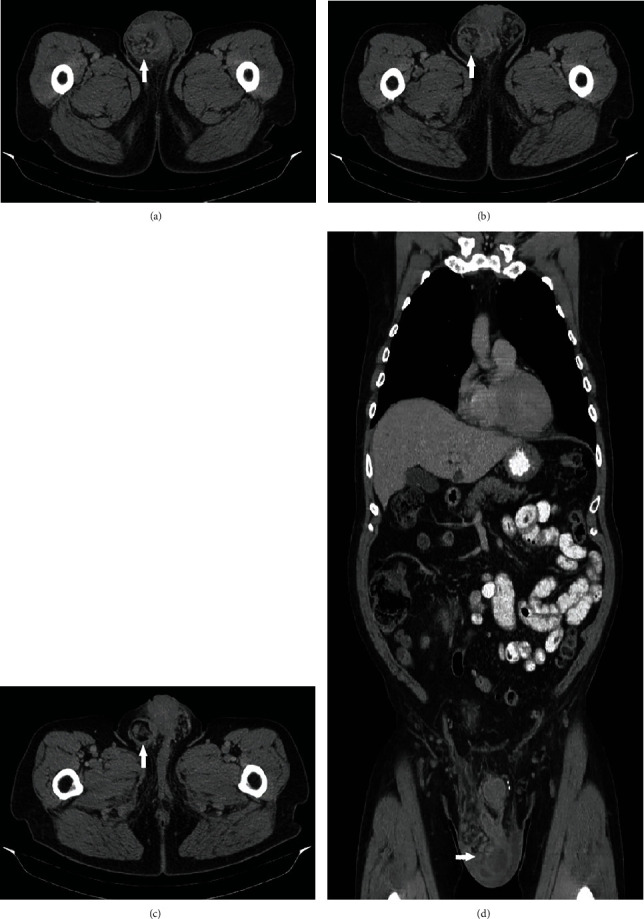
Computed tomography (CT) scan of the chest, abdomen, and pelvis noting a right-sided scrotal mass (white arrows) without signs of a primary tumor site or metastasis. Axial view (a-c) shows the scrotal mass (white arrow), and coronal view (d) shows the entire chest, abdomen, and pelvis.
